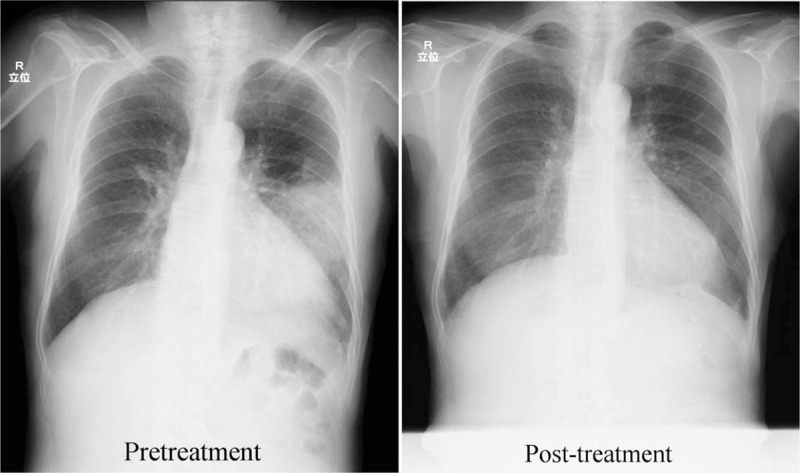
Chest x-ray. (A) On admission, a shadow was noted in the left middle over the lower lung field. (B) After treatment, the shadow was mostly resolved.
